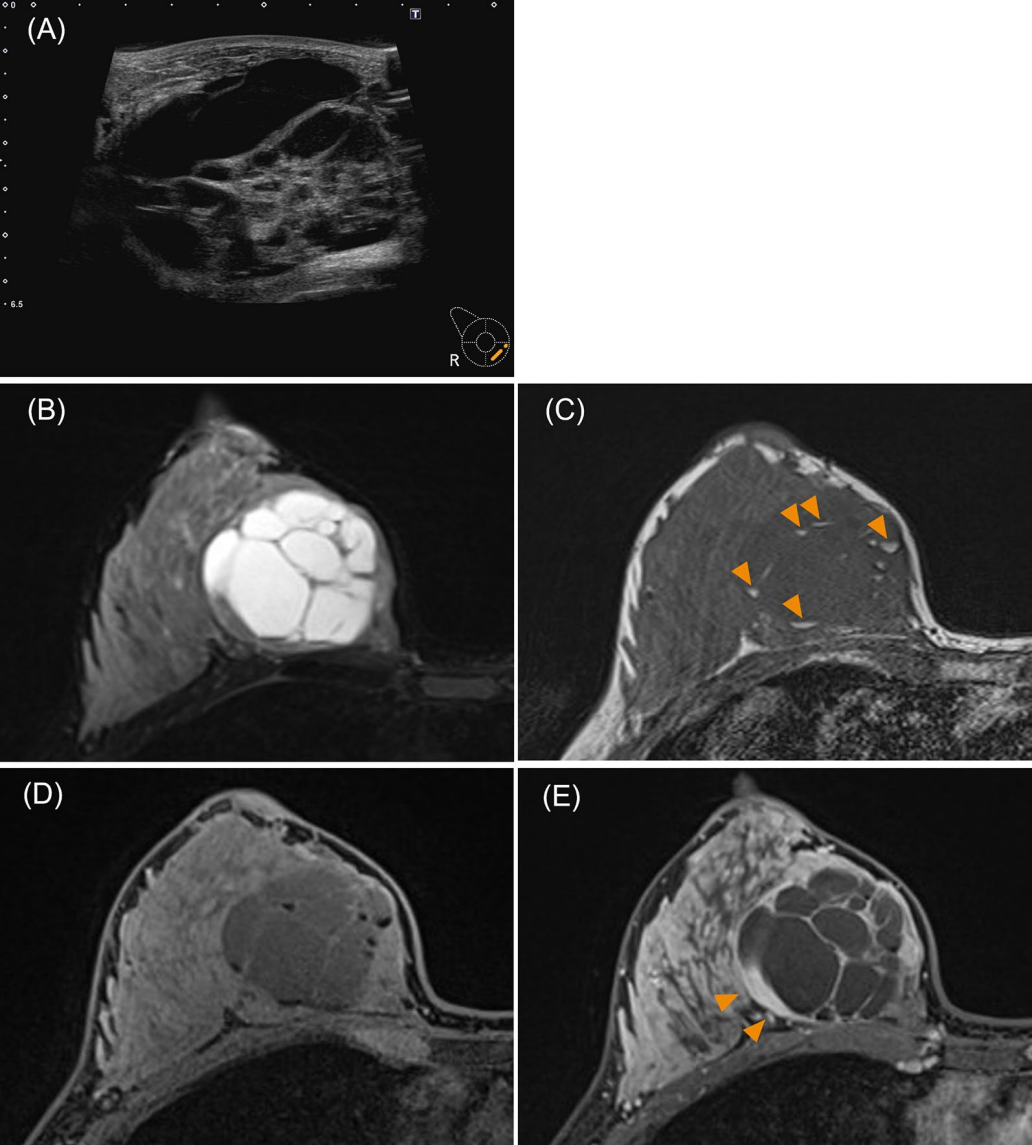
Findings of pre-surgical imaging. A Findings of ultrasound at the initial visit to our hospital (5 week postpartum). A well-defined lobulated mass with clear borders is observed in the right lower inner quadrant. Internal septations are present, and there is evidence of fluid retention. B–E Findings of the breast MRI performed 2 weeks before surgery (11 week postpartum). The tumor is exerting pressure outward, displacing the breast tissue. B T2-weighted image with fat suppression. The T1-weighted image (C) showed a high-signal area in the fluid component within the cyst (arrow), and this signal was suppressed in the fat-suppressed image (D). E Enhanced T1-weighted image with fat suppression in the late phase after contrast. An arrow indicates a solid part along the edge of the tumor
